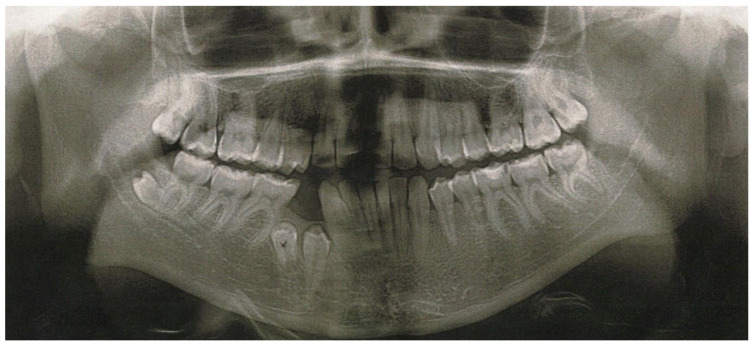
Panoramic radiograph, taken one year later, when patient was 16 years old, showing the further migration of the tooth 45—the germ was then located above the mandibular canal, distally from the roots of the tooth 48. The impacted tooth 44 and the third additional tooth had not changed their position since the previous X-ray picture had been taken. Furthermore, the crypt of the forming fourth additional tooth in the projection of 1/3 middle part of the root of the tooth 35 appeared.
